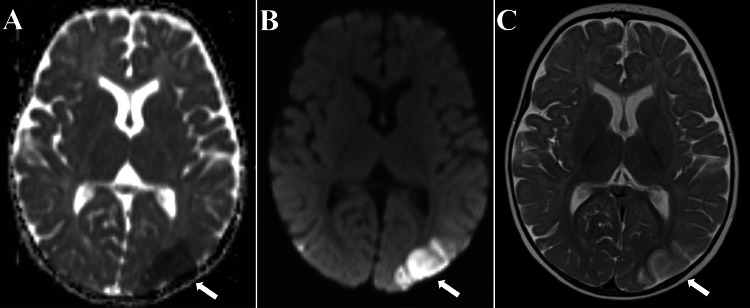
MRI consistent with infarcts of the left parietal lobe and temporo-occipital region with diffusion restriction (white arrows in panels A and B) as evidenced by the apparent diffusion coefficient (A) and diffusion-weighted imaging (B) sequences. This is likewise reflected by hyperintensity (white arrow in panel C) on T2-weighted imaging (C).
